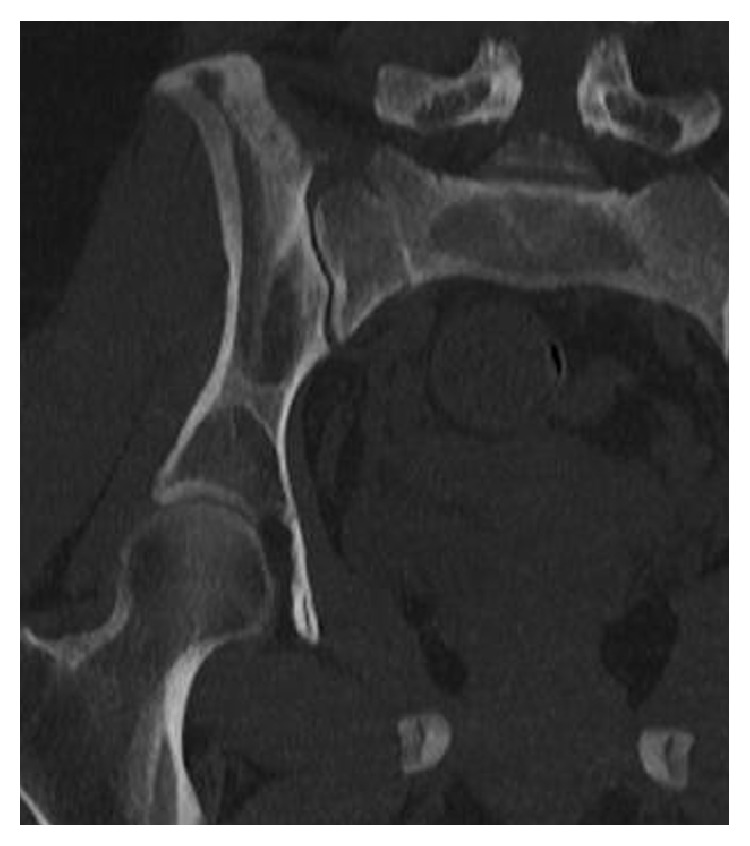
CT scan: coronal view of the right pelvis that showed the fracture line from the superior to the inferior border of the sacral bone with disruption of the cortical bone.
